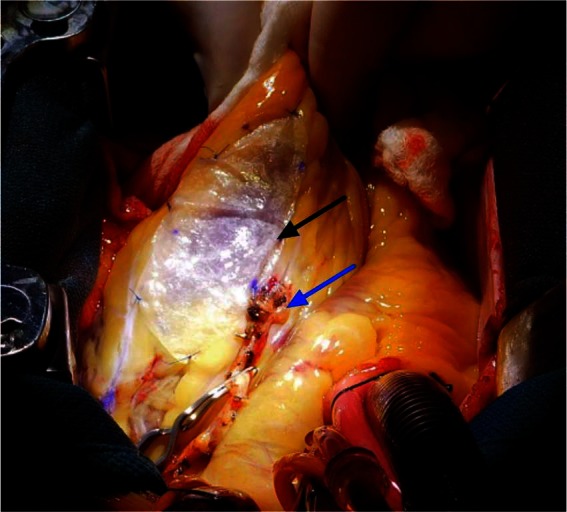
Representative image of surgical implantation of a bioactive scaffold at the time of revascularization surgery. Patients were selected for bioactive scaffold therapy in adjunct with CABG and followed by serial cardiac MRI up to six months following surgery. Black arrow indicates acellular bioactive scaffold. Blue arrow indicates bypass graft.
